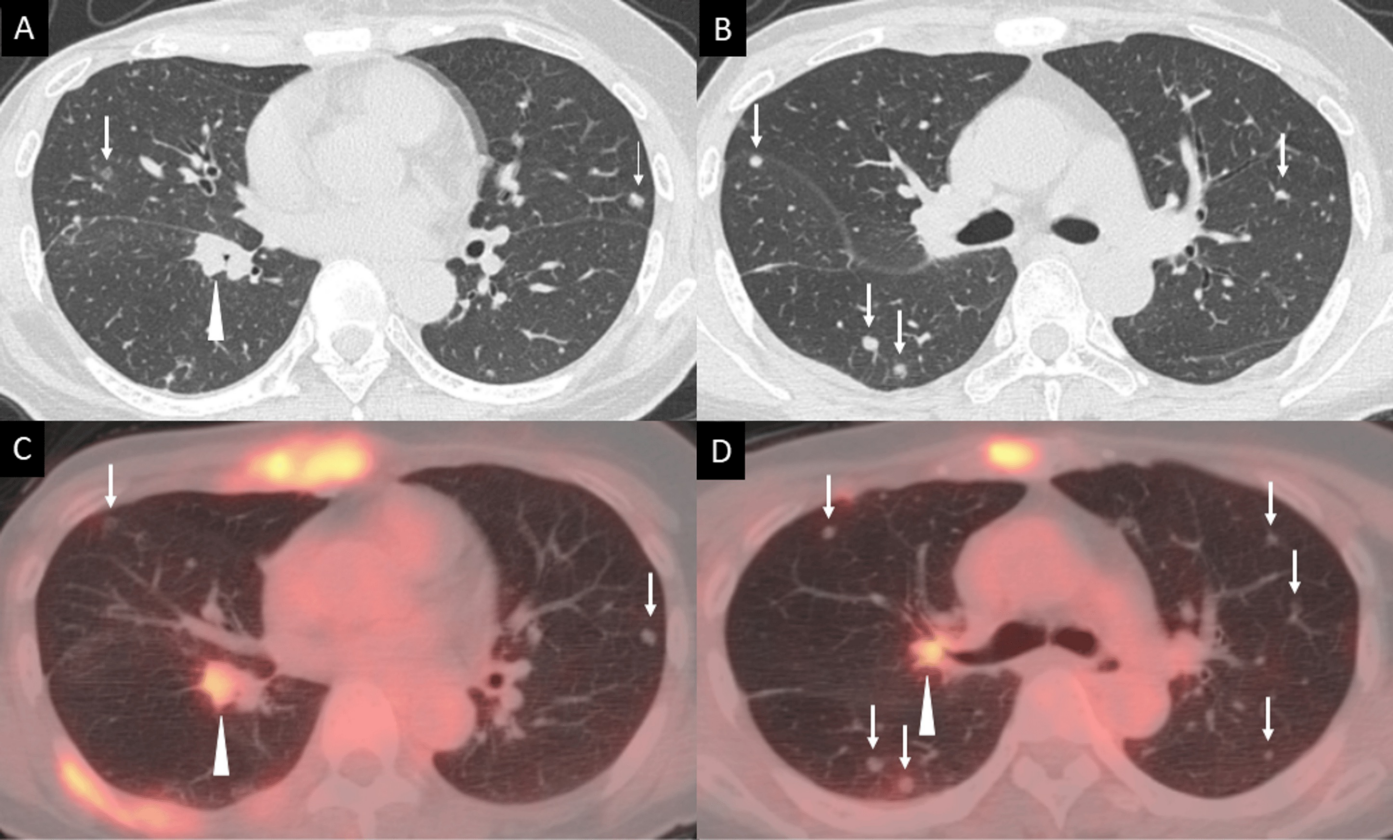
Chest CT and PET/CT findings of case 1 (A, B) Chest CT images showing multiple small pulmonary nodules measuring 2–5 mm in diameter (arrows) and right hilar lymphadenopathy (arrowheads). The right hilar lymph node (station 12R) is indicated in panel A. (C, D) PET/CT images demonstrating intense FDG uptake in the hilar lymph nodes (arrowheads) and sclerotic bone metastases, whereas the pulmonary nodules exhibit only mild uptake (arrows). CT, computed tomography; PET/CT, positron emission tomography/computed tomography; FDG, fluorodeoxyglucose
